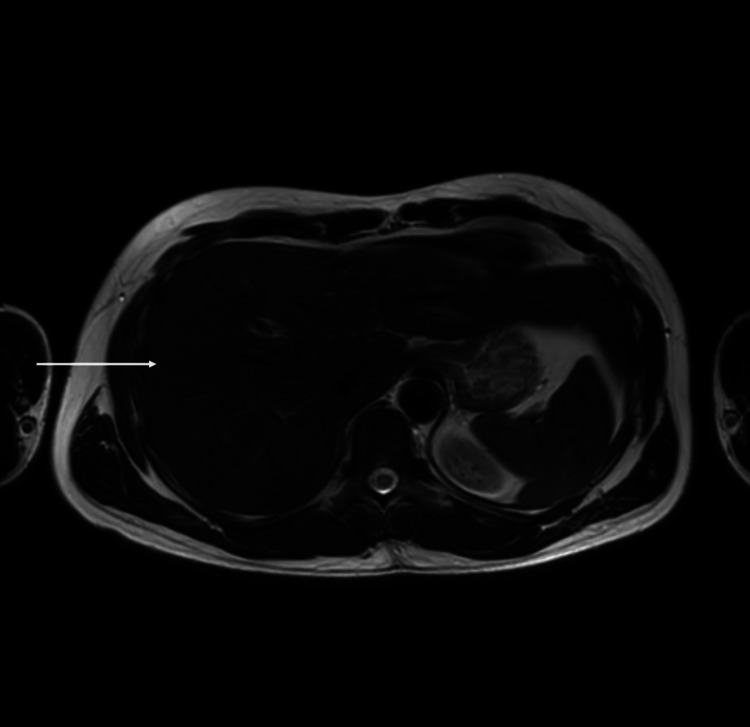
MRI of the abdomen. This study revealed mildly reduced T2 signal intensity in the liver with sparing of the spleen compatible with early primary iron deposition.
